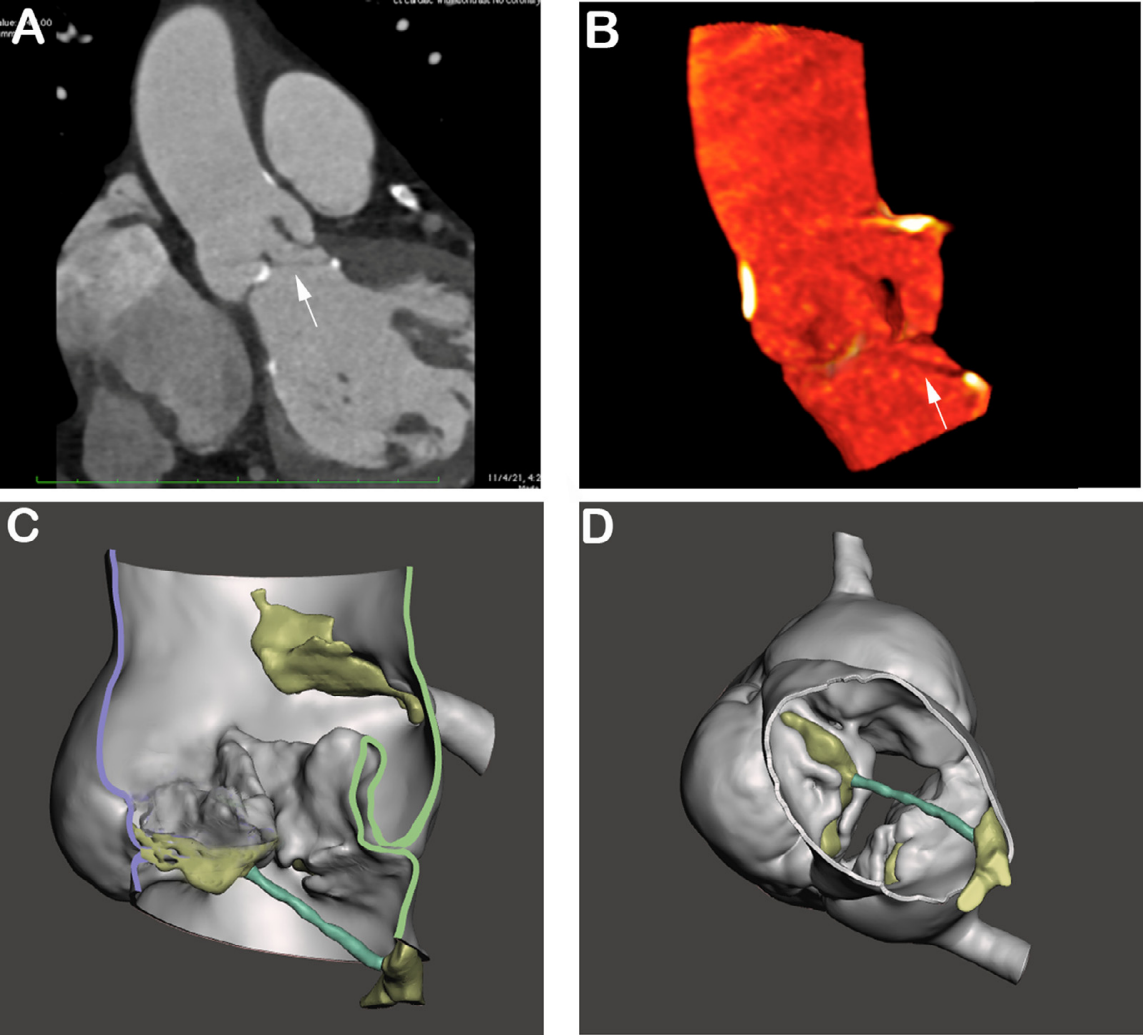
Cardiac CT of aortic valve chordae tendineae. Multi-planar reformatted image (A) and volumetric 3D display (B) of the LVOT and aortic root with a notably calcified aortic valve and fibrous strand (arrow) tethering the non-coronary cusp to left ventricular outflow tract. A right coronary cusp cut-away of a 3D STL display (C) shows a longitudinal view of chordae (teal) with aortic calcifications (yellow). A subvalvular projection of the aortic valve (D) visualizes the fibrous strand attachments to the non-coronary cusp and LVOT.
